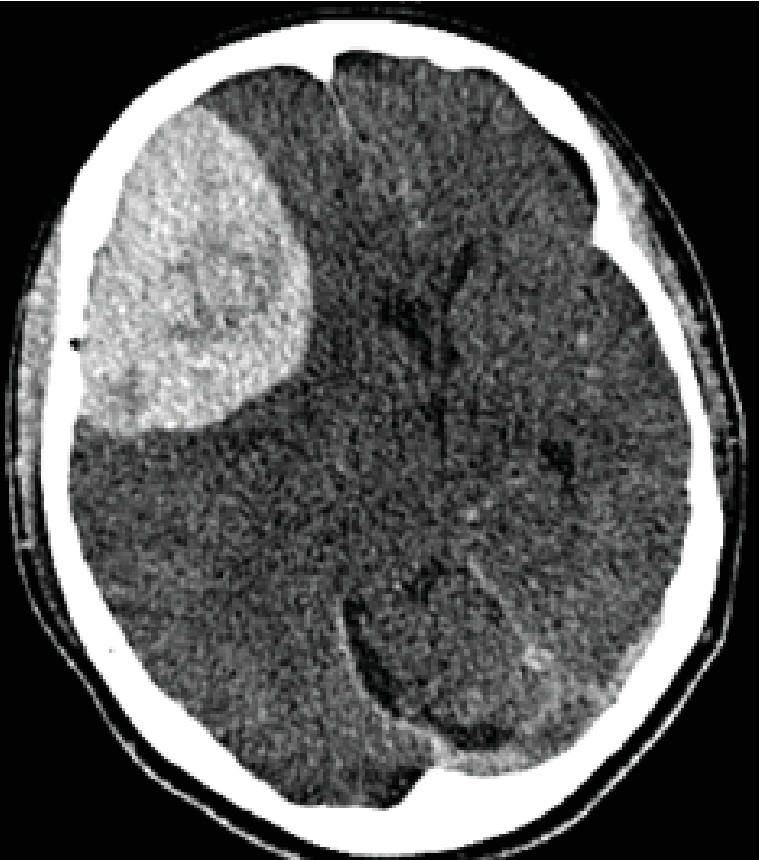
A head computed tomography (CT) scan showed a 90 × 90 mm mass in the right frontal lobe.
